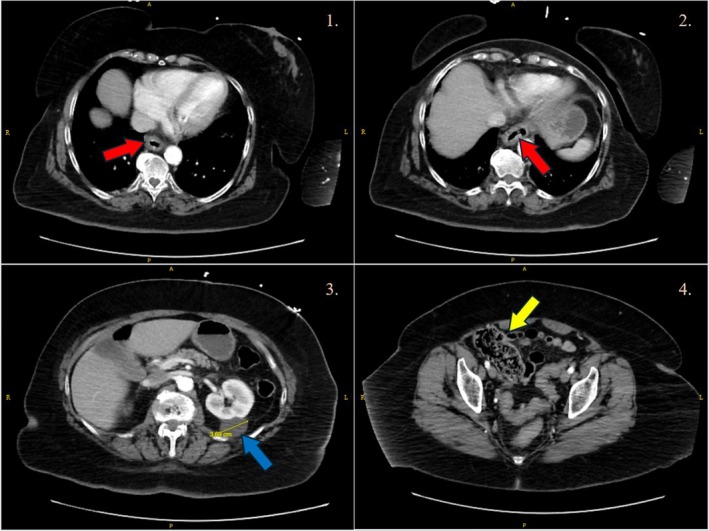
Contrast‐enhanced computed tomography of the abdomen and pelvis (axial sections) demonstrating wall thickening of the distal esophagus consistent with esophagitis (red arrows in panels 1 and 2), an incidental simple left renal cyst measuring 3.7 cm (blue arrow in panel 3), and uncomplicated sigmoid diverticulosis (yellow arrow in panel 4). No obstruction or intra‐abdominal infection was identified.
